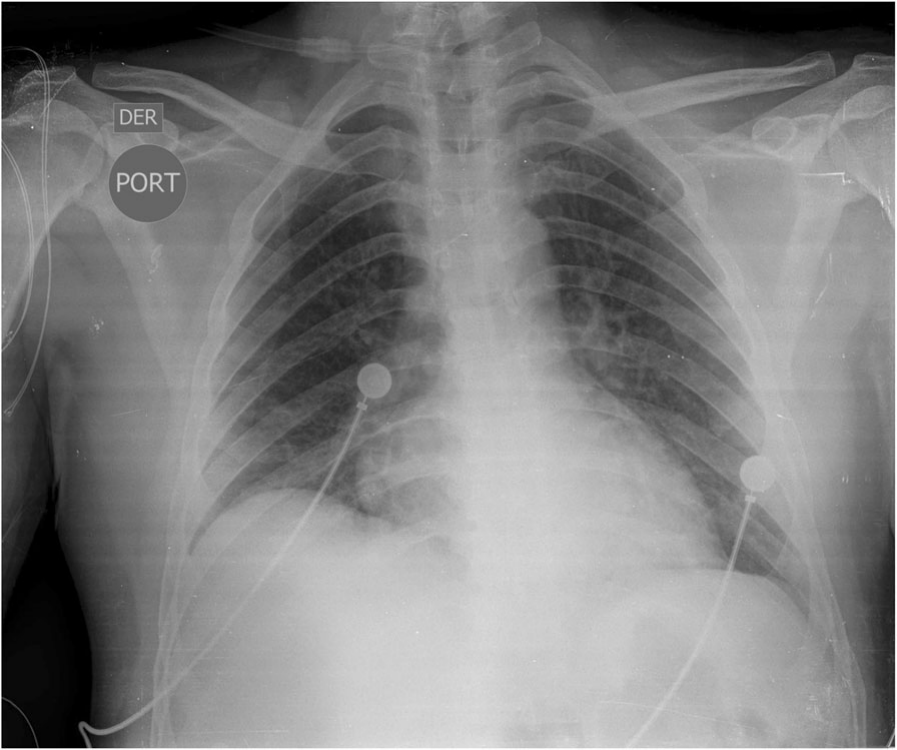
Chest X-ray acquired on admission of an adult patient with suspected pertussis
